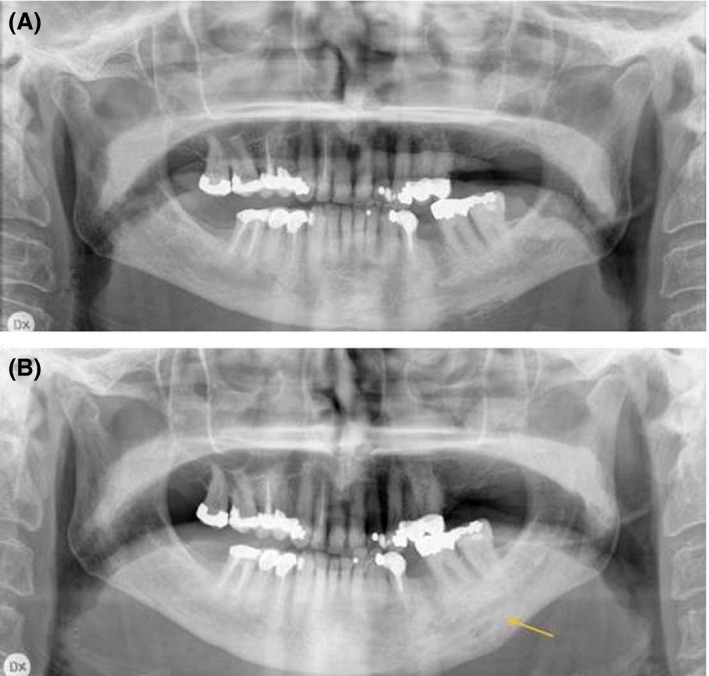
Radiological examinations of a 71‐year‐old woman with diffuse sclerosing osteomyelitis of the left side of the mandible for two years, treated with corticosteroids and clindamycin for two years. A, Orthopantomogram before treatment with denosumab revealing sclerosis, resorption, and periosteal apposition of the left side of the mandible. B, Orthopantomogram taken after 12 mo with denosumab treatment showing less radiolucency and maturation of the bone in the area of periosteal apposition
